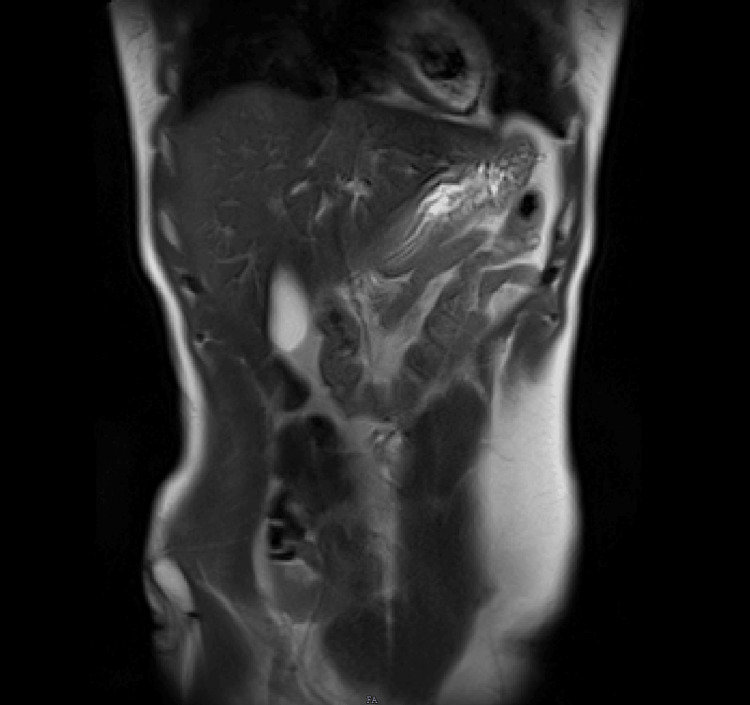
Coronal T2-weighted MRI sequences demonstrating normal signal intensity and morphology of the visualized structures, with no evidence of focal lesions or abnormal fluid collections.
